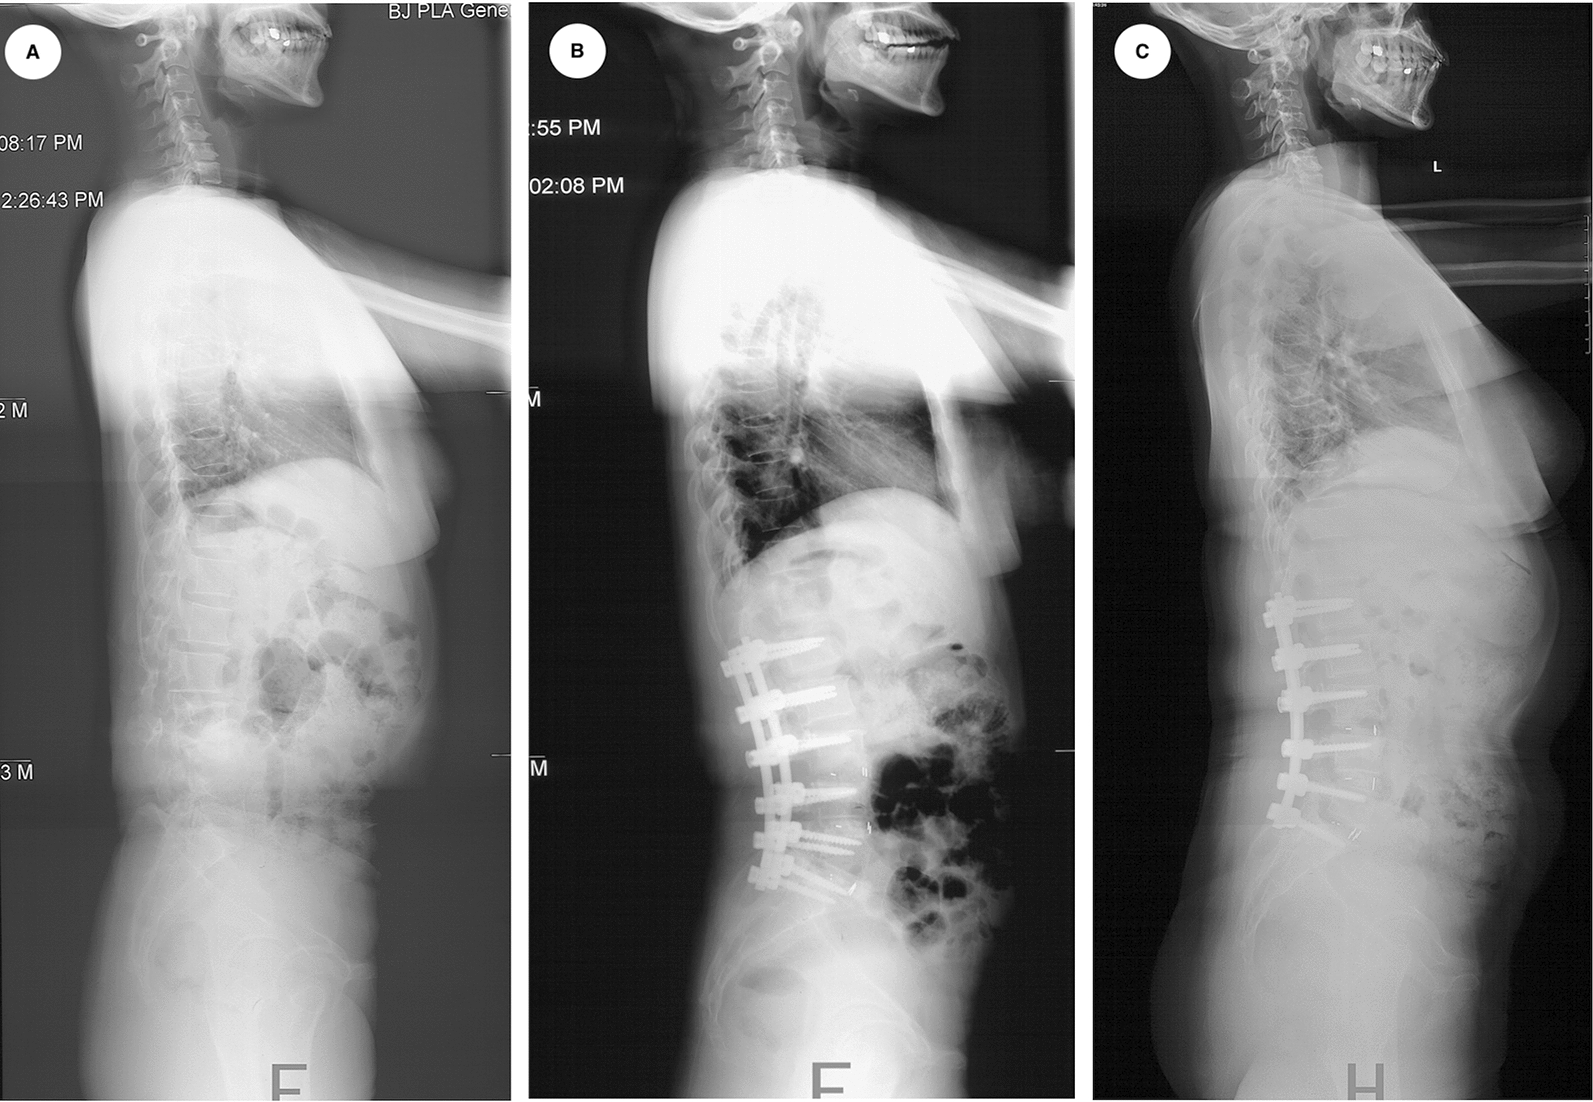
A 68-year-old male DLS patient underwent lumbar fusion surgery (L1–S1). Radiographs show the changes in spinopelvic parameters, preoperative TK, LL, PT, SS, PI, PFA, SAA, SVA and TPA were 6.6°, −15.8°, 26.6°, 25.4°, 52.0°, 200.0°, 36.0°, 2.6 mm and 17.7°, respectively (A). Postoperatively, those variables were 14.7° for TK, −41.1° for LL, 18.8° for PT, 34.1° for SS, 52.9° for PI, 185.4° for PFA, 35.9° for SAA, −27.3 mm for SVA, and 8.7° for TPA (B). At the final follow-up, those variables were 15.8° for TK, −41.2° for LL, 18.9° for PT, 38.9° for SS, 57.8° for PI, 189.6° for PFA, 36.2° for SAA, 17.8 mm for SVA, and 14.6° for TPA (C). The PI–LL was 36.2°, 11.8° and 16.6° at the pre-, post-operation and the final follow-up, respectively
